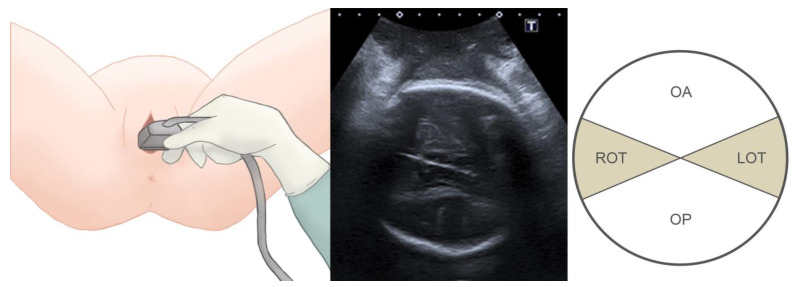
(Left) Transperineal ultrasonography to evaluate the fetal head position. (Center) Visualization of the midline angle plane to assess the fetal head position. (Right) Classification of the fetal occiput positions (OA: occiput anterior; LOT: left occiput transverse; OP: occiput posterior; ROT: right occiput transverse).
